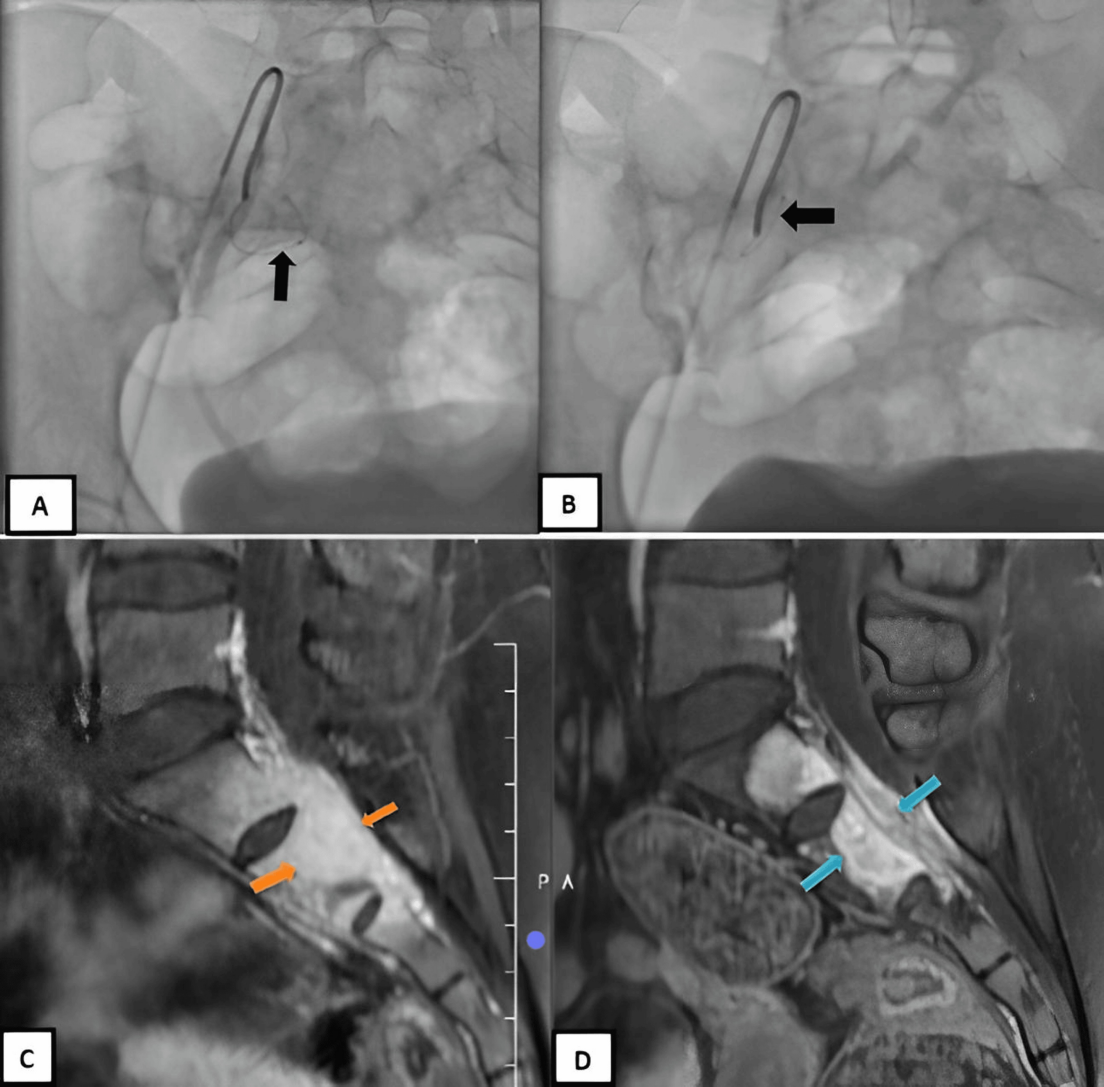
DSA AP view (A, B): Selective angioembolization of branches of the right internal iliac artery supplying the tumor. The branches were individually cannulated (black arrow) and embolized using 300-500 u PVA particles. (C, D) MRI Sag T1 FS + C before and after denosumab therapy and angioembolization revealed reduction in the AP diameter (orange and blue arrows) of the lesion with reduction of the cauda equina compression but the same contrast enhancement as above, suggesting partial resolution.
